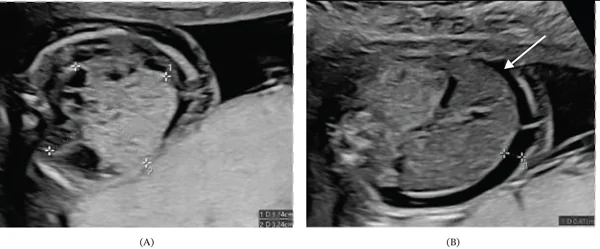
Ultrasound examination at 17 + 6 weeks. (A) An increase in the size of the mass in the right hemithorax (D1: latero‐lateral diameter; D2: anteroposterior diameter). (B) Worsening of ascites (D1) and skin edema (white line).
